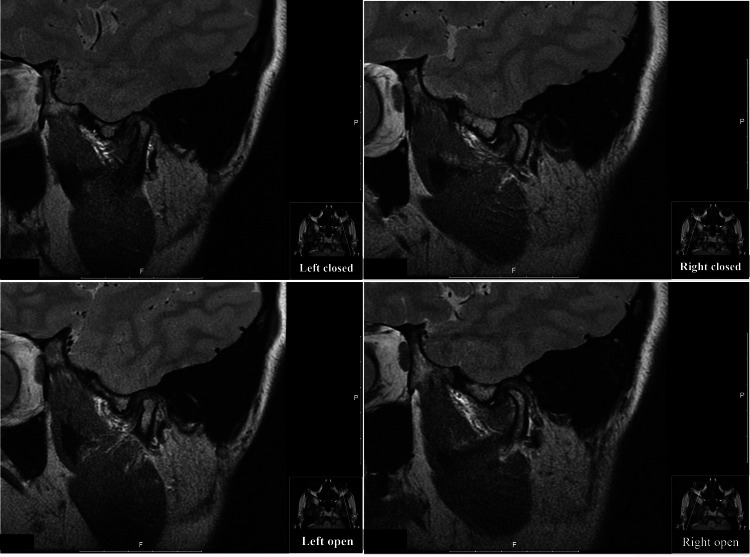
Magnetic resonance imaging of the left and right TMJ/temporal fossa regions. Sagittal closed mouth views (upper panels); sagittal open mouth views (lower panels). TMJ: temporomandibular joint.
